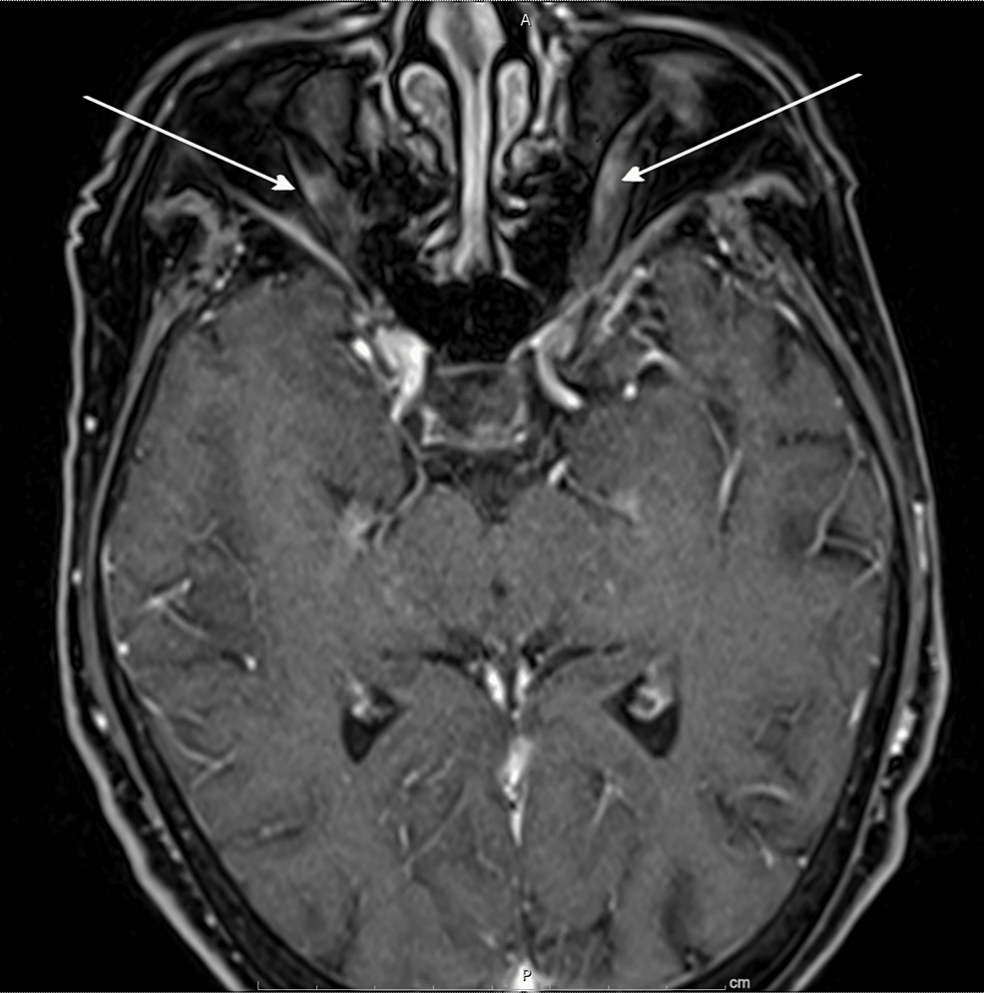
Patient’s head MRI with abnormal enhancement of optic nerves. MRI: magnetic resonance imaging.
